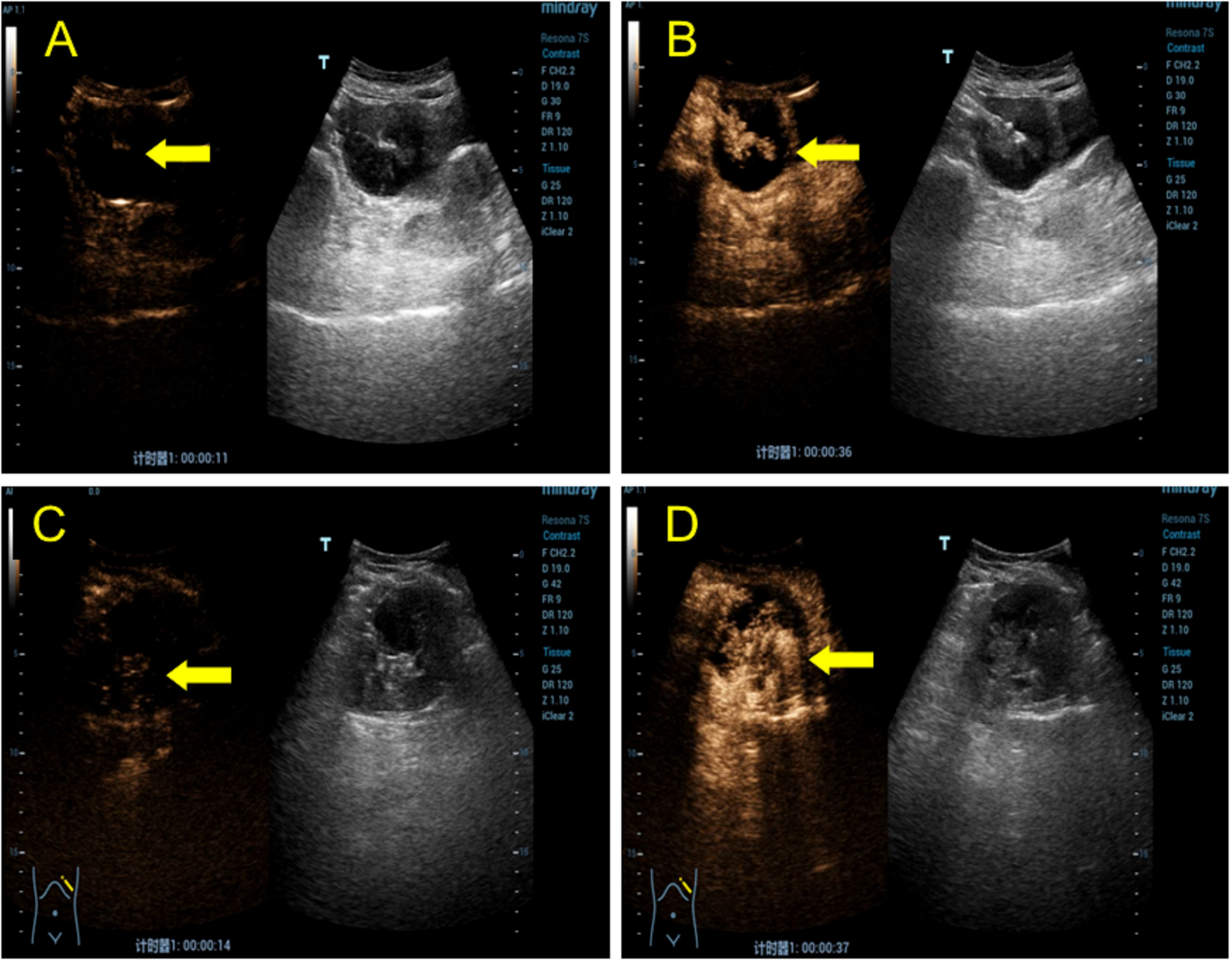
CEUS shows a lesion in the right lower abdomen and spleen. (A) In the transabdominal contrast-enhanced ultrasound examination, the solid capsular part of the mass in the right lower abdomen began to perfuse at 11 s (yellow arrow). (B) Isoenhancement is seen in the center (yellow arrow), and no contrast perfusion is observed in the cystic portion. (C) During the transabdominal contrast-enhanced ultrasound examination, the mixed mass of cystic and solid capsulated in the spleen began to perfuse at 14 s (yellow arrow). The contrast agent was unevenly distributed, with visible perfusion in the solid part but not in the cystic part. (D) The contrast agent entered the solid part (yellow arrow), which was isoenhanced, and the enhancement subsided at 37 s, which was earlier than that of the normal spleen tissue.
